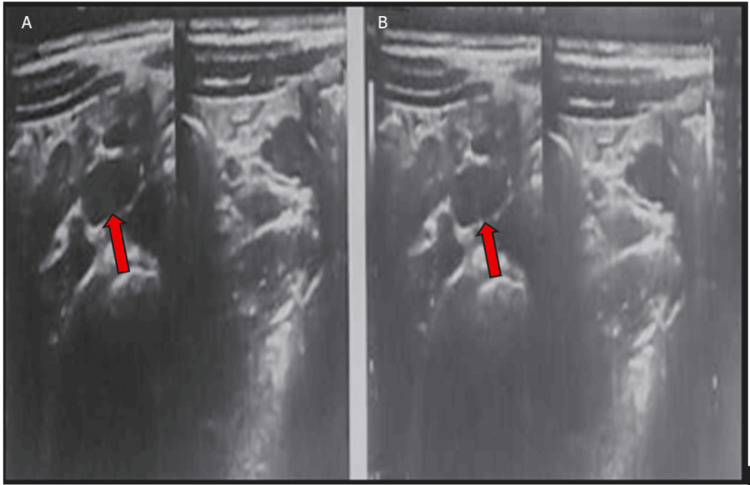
(A, B) Abdominal ultrasound images with the red arrows indicate dilated bowel loops containing echogenic foci of gaseous distension.
